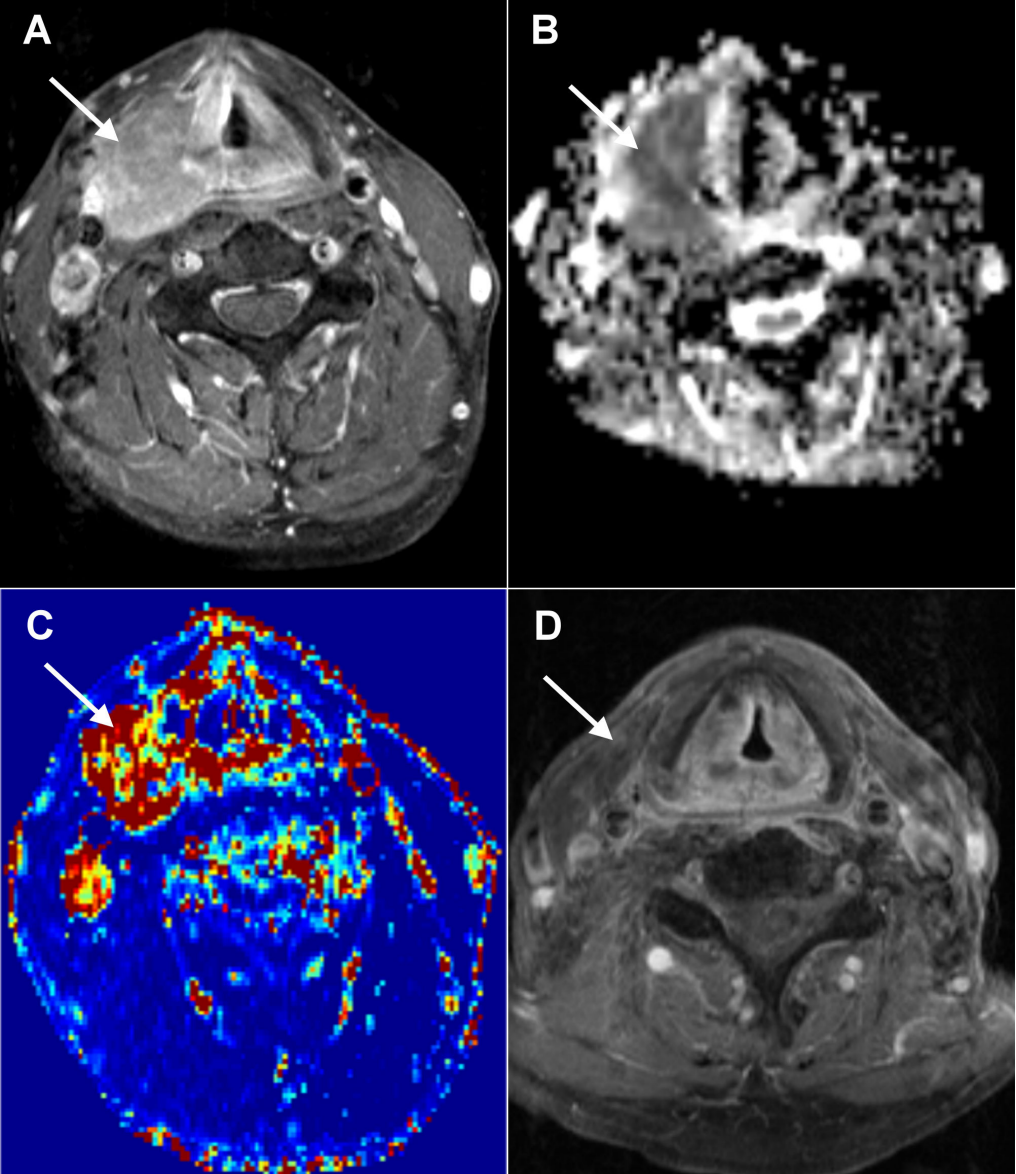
Local control in a 52-year-old man with hypopharyngeal SCC after chemoradiation. A. A pretreatment axial-enhanced MRI shows the right hypopharynx tumor (arrow). B. The corresponding PWI map shows the tumor with a *K*
